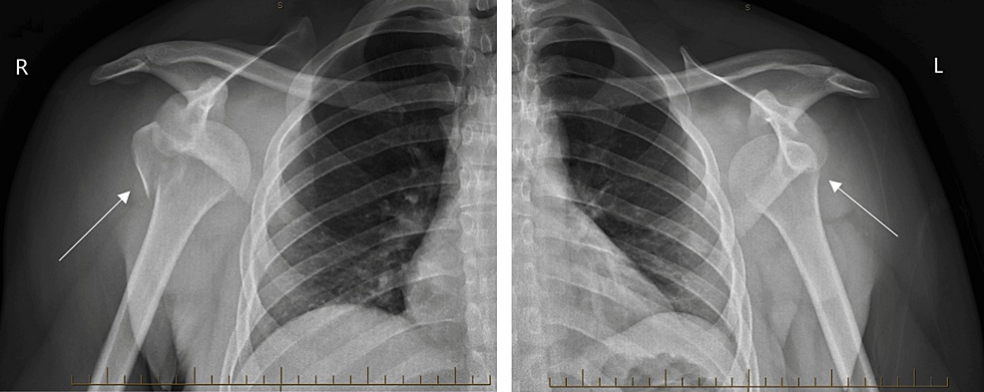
Bilateral shoulder X-rays showing bilateral anterior dislocations. There is also a fracture through the greater tuberosity of the right humerus.
